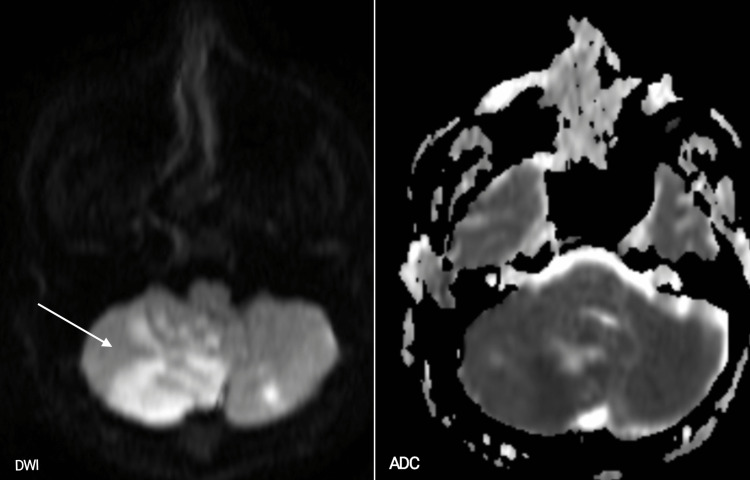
DWI MRI Image shows restriction (arrow) corresponding to low signals on ADC.
